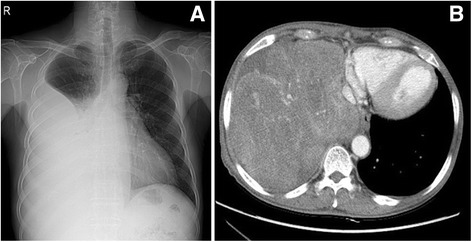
Chest radiograph on surgical treatment period. (a) Shows the increasing mass shadow occupying middle and lower zone of right hemithorax. (b) Chest CT during evaluation of lung mass shows lobulated heterogenously enhancing mass in right lower lobe lung field
